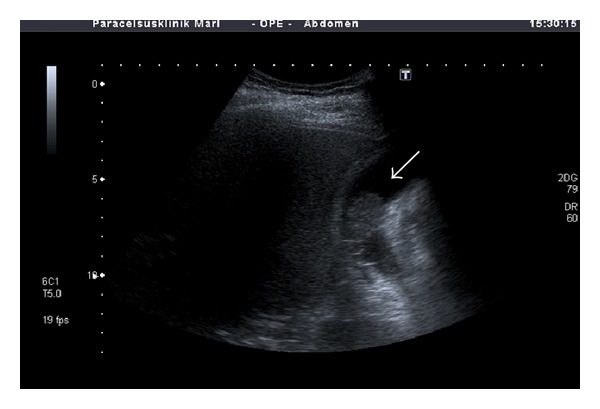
B-mode sonography showing a 25 mm polypoid lesion within the gallbladder (arrow). The tumor appears to have broad contact to the gallbladder wall at two locations.
